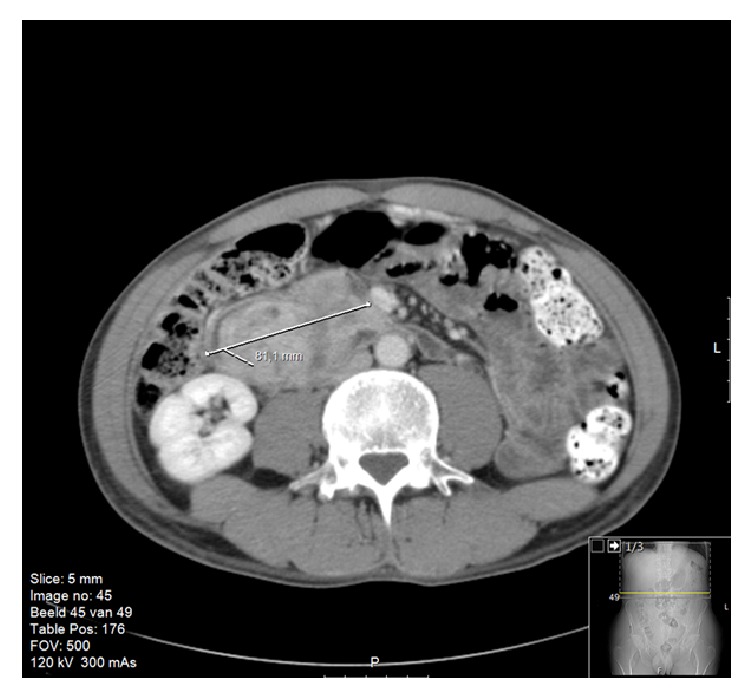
Axial CT view showing a large mass of approximately 8 cm in diameter, near the proximal duodenum. Oral and IV contrast were given.
